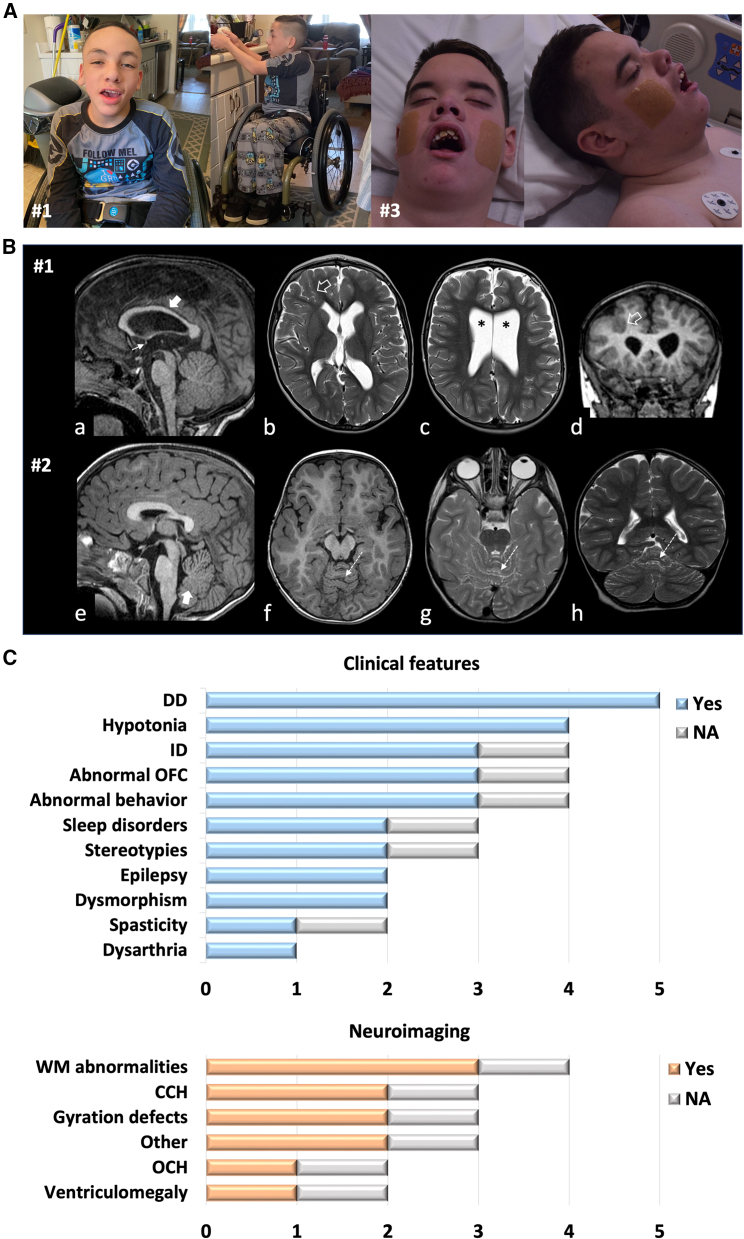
Clinical and neuroimaging details of subjects harboring *DENND5B* variants (A) Clinical photographs of subjects harboring *DENND5B* variants. Subject #1 shows macrocephaly, mild intellectual disability, axial hypotonia, and appendicular spasticity. Facial dysmorphism includes arched and sparse eyebrows, narrow palpebral fissures, hypertelorism, bulbous nose tip, deep nasal bridge, thick lower lip, and prominent chin. Subject #3 has severe cognitive deficiency, microcephaly, and hypotonia. Dysmorphic features include short nose, hypertelorism, malposition of teeth, deep nasal bridge, deep philtrum, and micrognathia. (B) Neuroimaging findings. (a–d) Brain MRI of subject #1 with sagittal T1-weighted (a), axial T2-weighted (b, c), and coronal T1-weighted (d) showing a thin corpus callosum (thick arrow), a small anterior commissure (thin arrow), white matter volume loss with ventricular enlargement (asterisks), and a focal gyral anomaly with infolding in the right frontal lobe (empty arrows). (e–h) Brain MRI of subject #2 with sagittal T1-weighted (a), axial T1-weighted (b), axial T2-weighted (c), and coronal T2-weighted (d) revealing focal foliar anomaly in the superior cerebellar vermis (dashed arrows) associated with inferior vermis hypoplasia (thick arrow). (C) Histogram graphs showing the distribution of clinical features and brain MRI abnormalities in the cohort of subjects harboring *DENND5B* variants. Abbreviations: CCH, corpus callosum hypoplasia; DD, developmental delay; ID, intellectual disability; NA, not available; OCH, optic chiasm hypoplasia; OFC, occipito-frontal circumference; WM, white matter.
